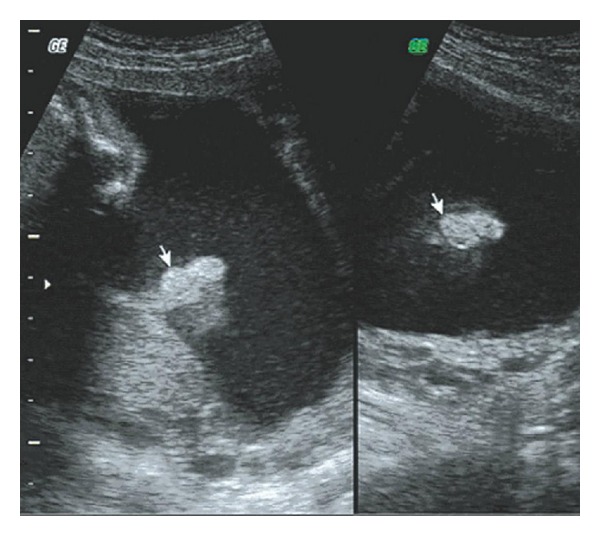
Sonogram of bilateral microtia on auricular parasagittal section.
